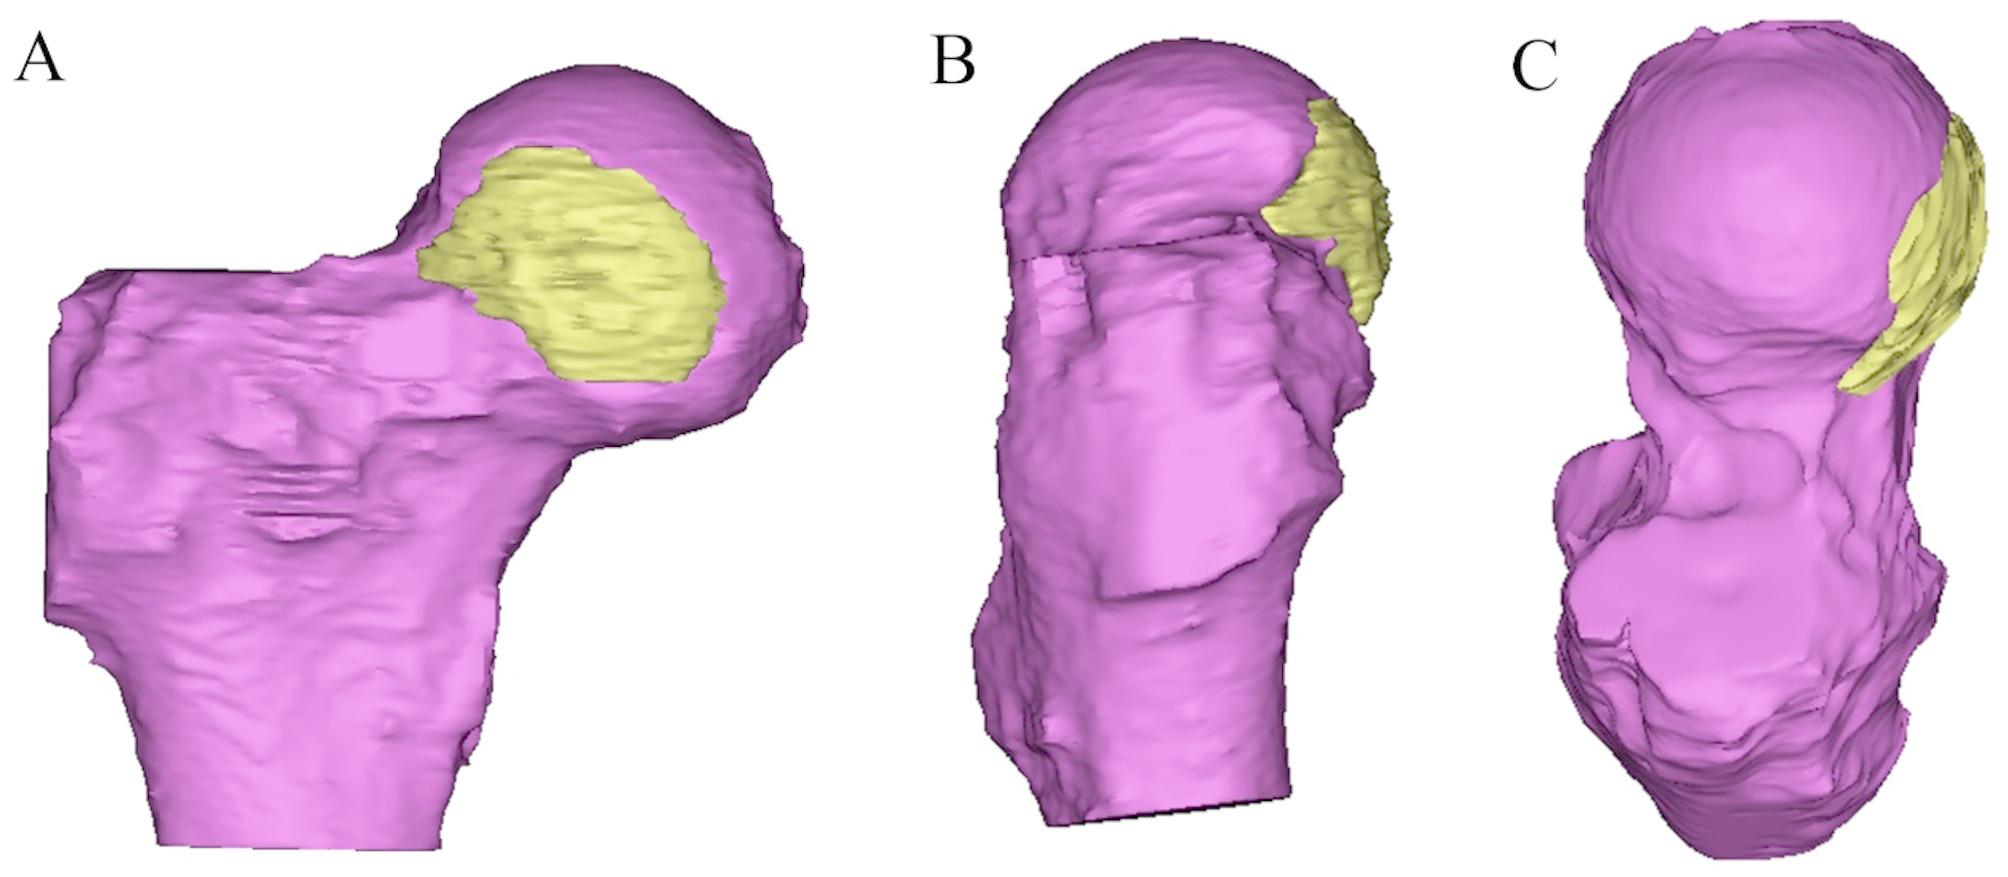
MRI reconstruction of FHI area. Note. One patient underwent MRI 3D reconstruction to enhance visualization of the FHI location. All FHIs were located in the anterior region of the femoral head. (A) Viewing from the front. (B) Viewing from the lateral side. (C) Viewing from above. Yellow area showed the range of FHI. Red area showed the normal femoral head. FHI, femoral head infarction
